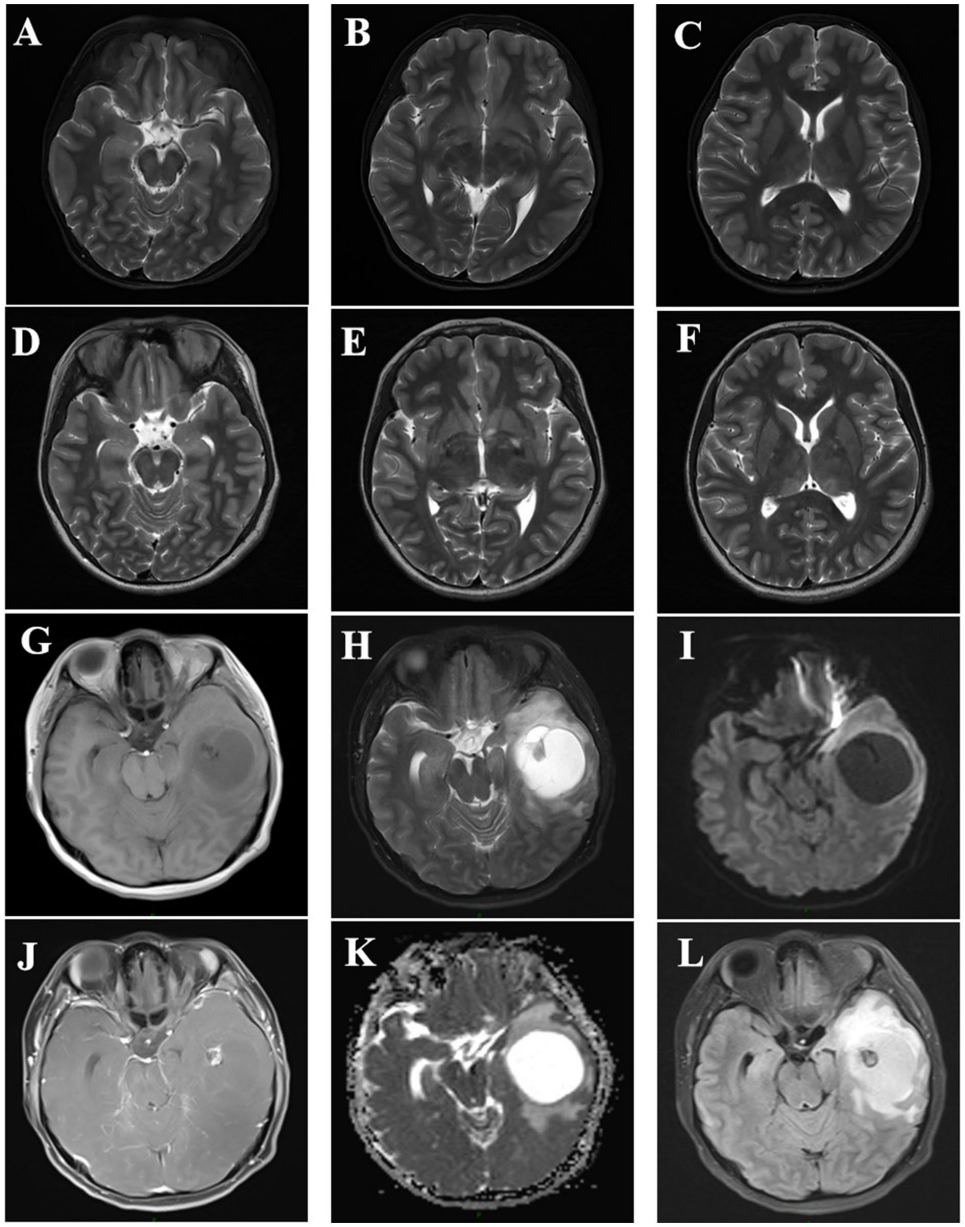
(A–C) Cranial magnetic resonance imaging of the patient at 11 years of age, showing patchy T2-weighted imaging (T2WI) high signals with fuzzy boundaries in the bilateral basal ganglia, thalamus, brainstem, and bilateral cerebellar hemispheres, with no diffusion limitation on diffusion-weighted imaging (DWI). (D–F) Cranial magnetic resonance results of the patient at the age of 14 years after a seizure, showing findings consistent with the previous results (A–F correspond to the same levels). G–L Cranial magnetic resonance imaging of the patient’s mother at the age of 31 years, showing changes indicating a solid cystic mass in the left temporal lobe. A T1-weighted imaging (T1WI), B T2WI, C apparent diffusion coefficient (ADC), D T1WIC+, E DWI, and F fluid-attenuated inversion recovery (FLAIR).
